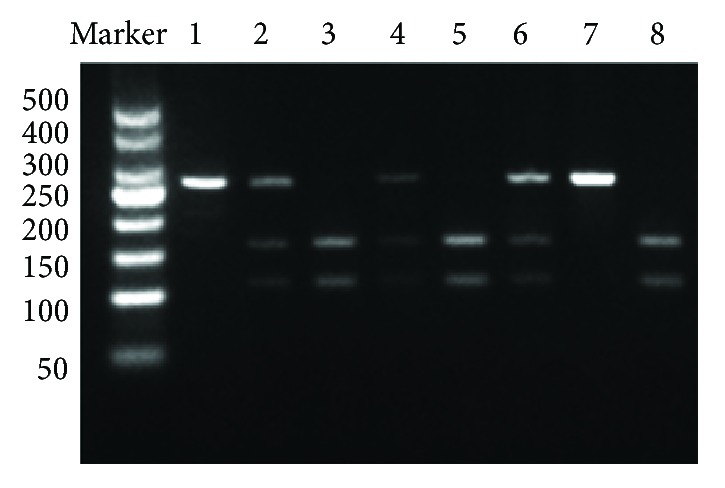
Agarose gel electrophoresis of the rs4612666 polymorphism. Line 1: TT; line 2: CT; line 3: CC; line 4: CT; line 5: CC; line 6: CT; line 7: TT; line 8: CC.
